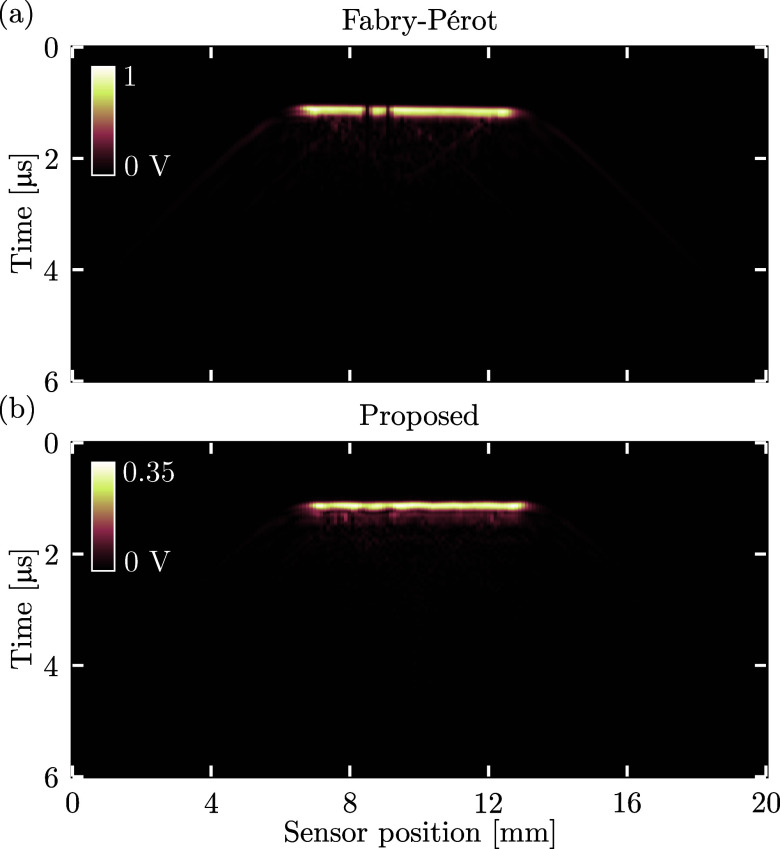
Visualisation of continuous ultrasound recording (“B-scan”) during motorised linear motion across the surface of an ultrasound source, using (a) a fibre-optic Fabry–Perot or (b) proposed non-resonant sensor.
